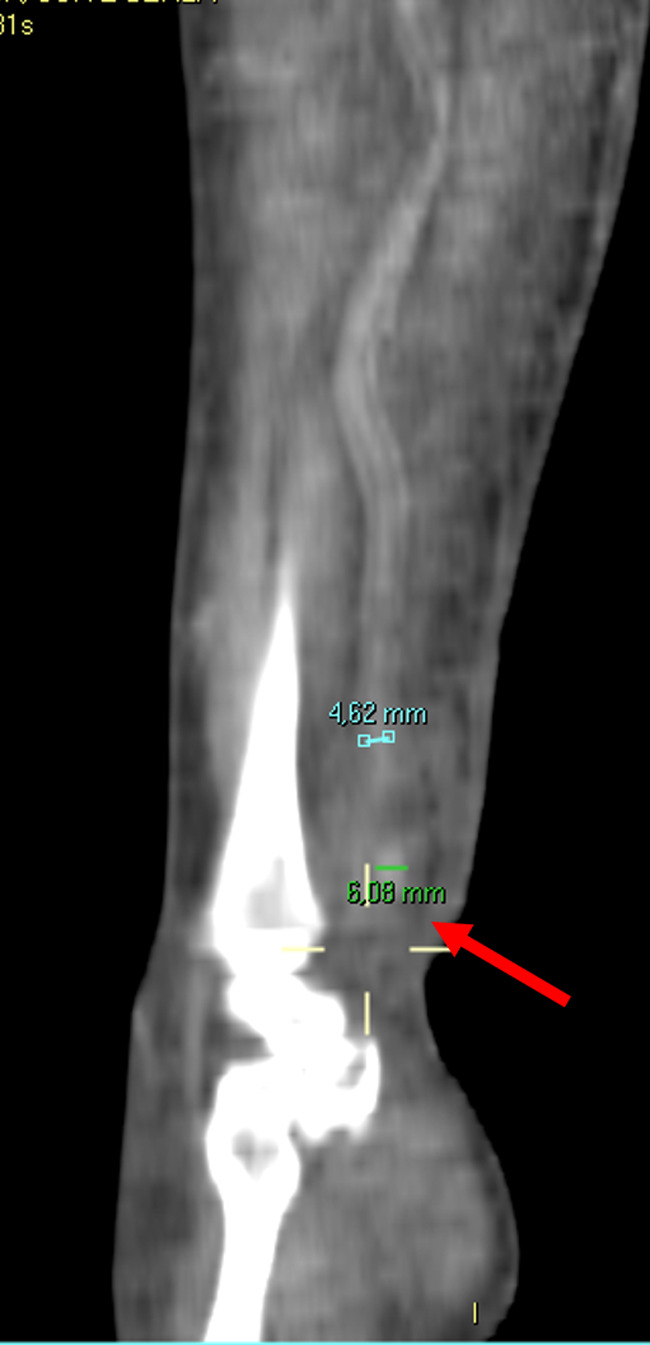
Right upper limb CTA showing a focal ectasia of the radial artery of 6 mm (red arrow), with edematous infiltration of the surrounding tissue and inflammation of the flexor muscles of the forearm
